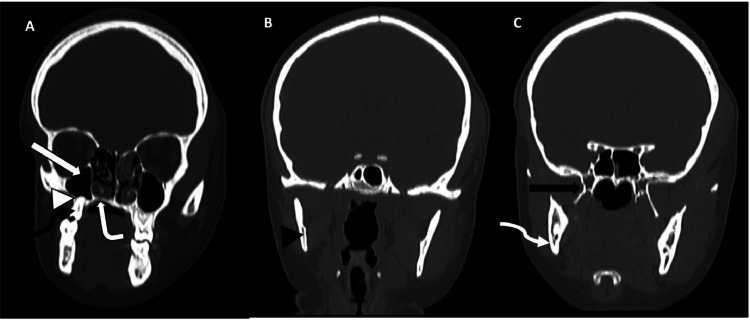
(A-C) Coronal reformatted CT scans of the head (bone window) shows reduction in the sizes of the palatine process (*white bent arrow*) and the alveolar process (*white arrowhead*) of the right maxilla with a smaller right maxillary sinus (*white block arrow*) and first right upper molar (*black squiggle arrow*) (A). There is also reduction in the size of the right mandibular ramus (*black arrowhead*) (B), the right mandibular angle (*white squiggle arrow*), the body, medial and lateral processes of the right pterygoid bone (*black block arrow*) (C).
